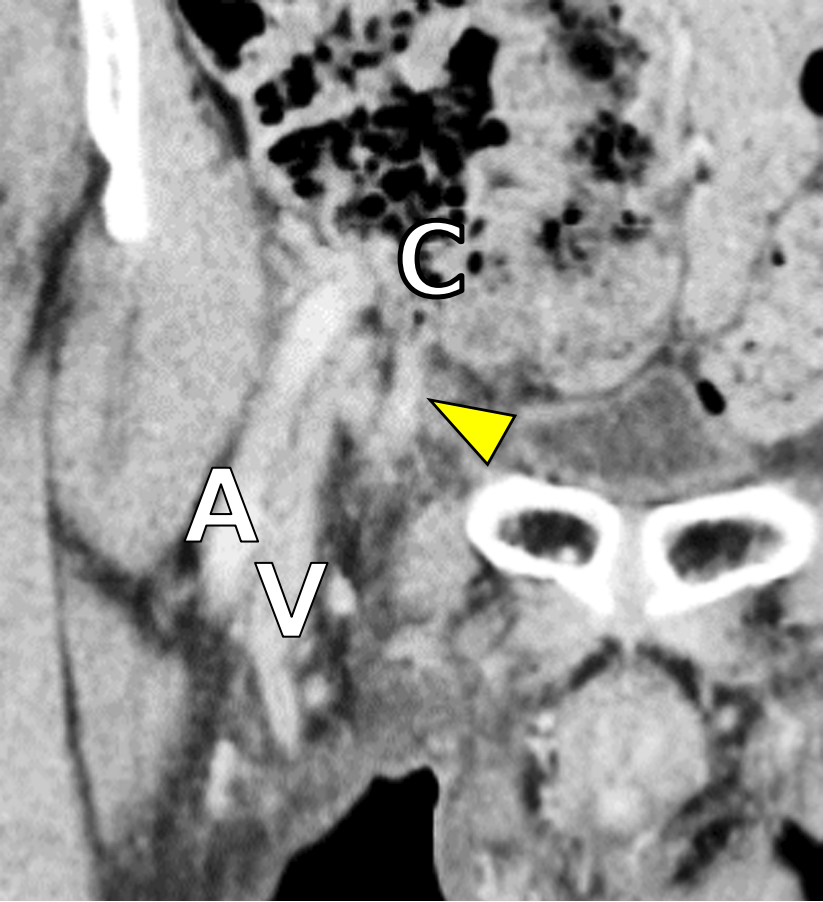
Coronal image of contrast enhancement CT showing a blind-ended tubular structure (arrowhead) on the medial side of the femoral vein, which is continuous with the cecum (C). A: femoral artery, V: femoral vein
